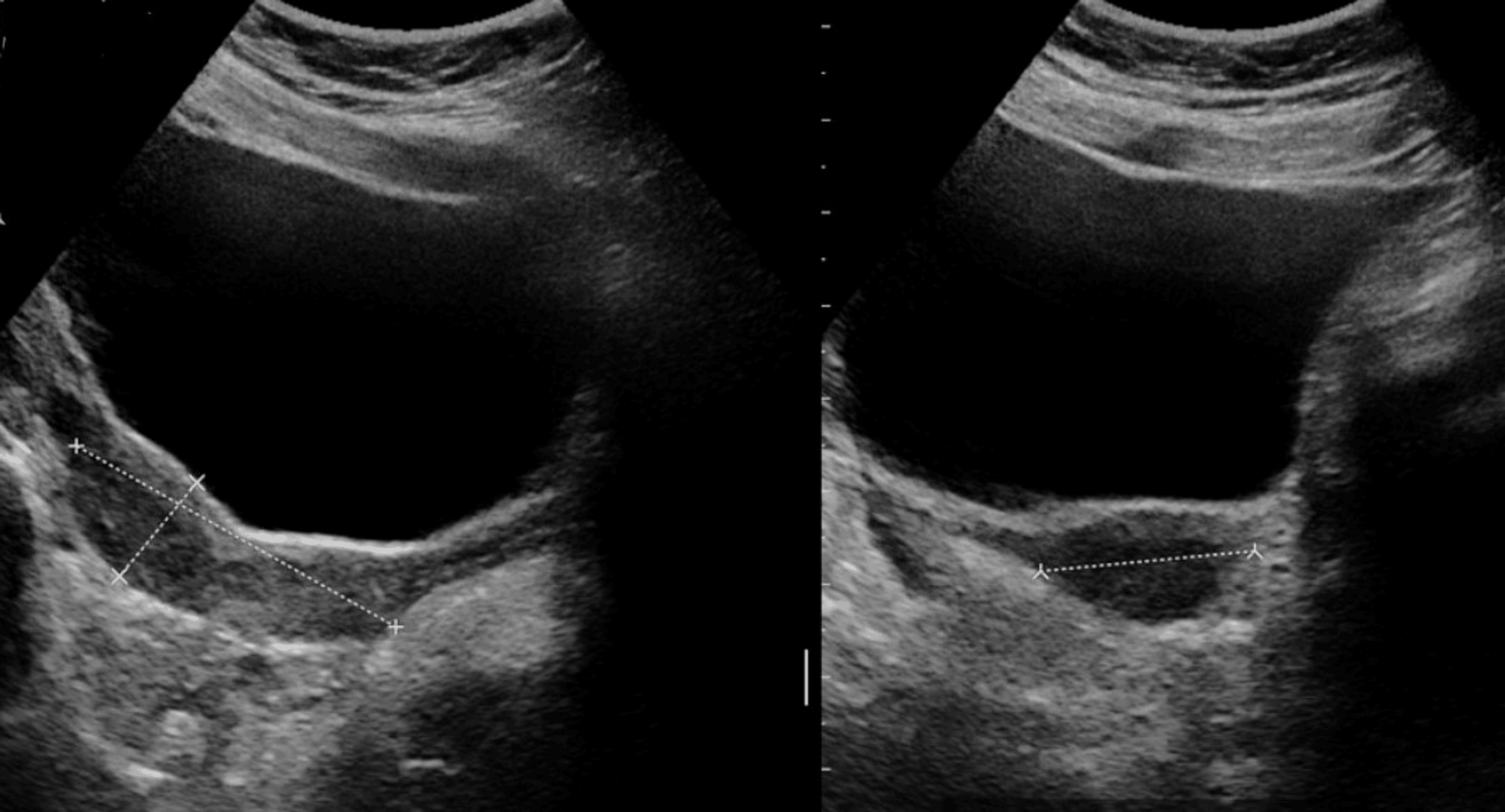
Pelvic ultrasonography showing normal morphology (uterus)
